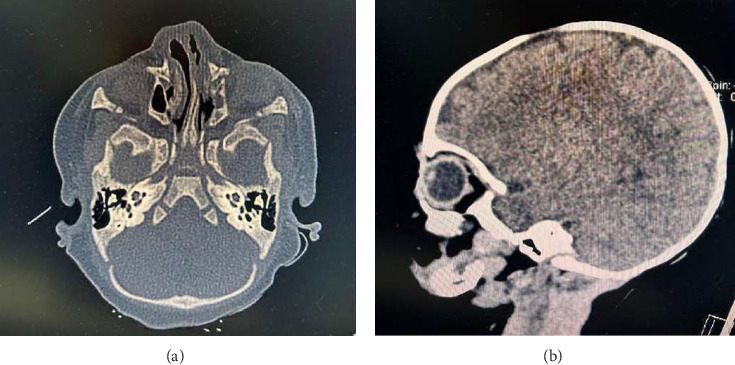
Coronal (a) and sagittal (b) computed tomography (CT) scans of the craniofacial skeleton.
